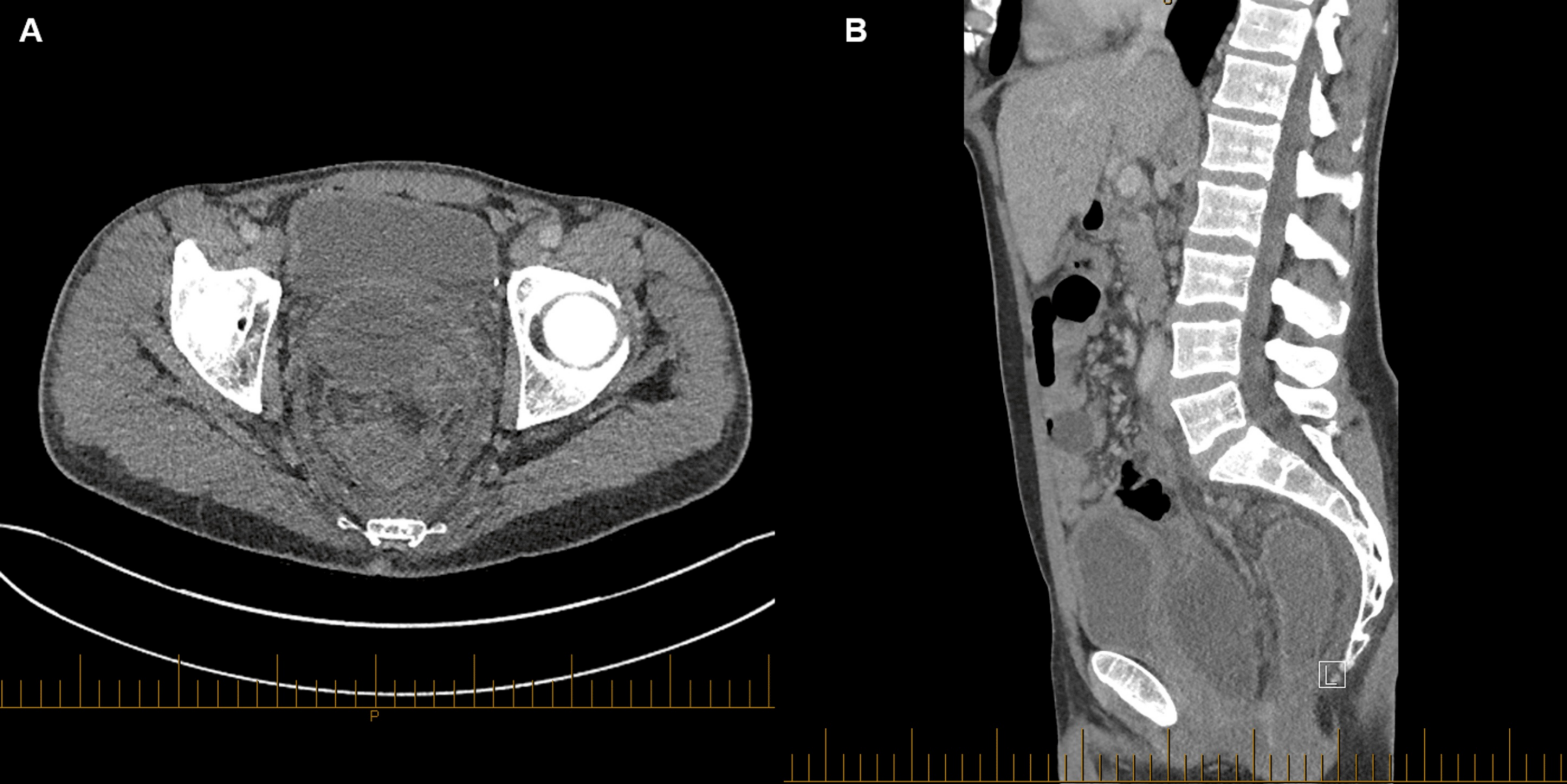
CT of the abdomen and pelvis 13 days after the Altemeier procedure. (A) axial, (B) sagittal, demonstrating an encapsulated infected collection evidenced by the thickened, enhancing peripheral wall and adjacent inflammatory fat stranding.
